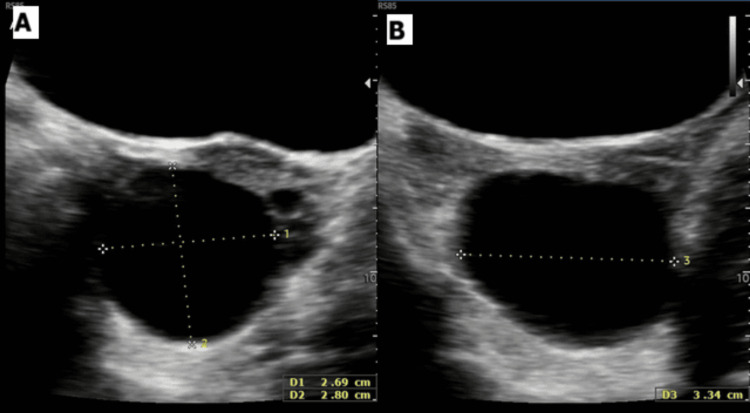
(A) Ultrasound demonstrating stable appearance of left ovarian simple cyst at four weeks post-op. (B) Longitudinal view confirming a thin-walled cyst with anechoic contents with a maximum diameter of ~3.3 cm
